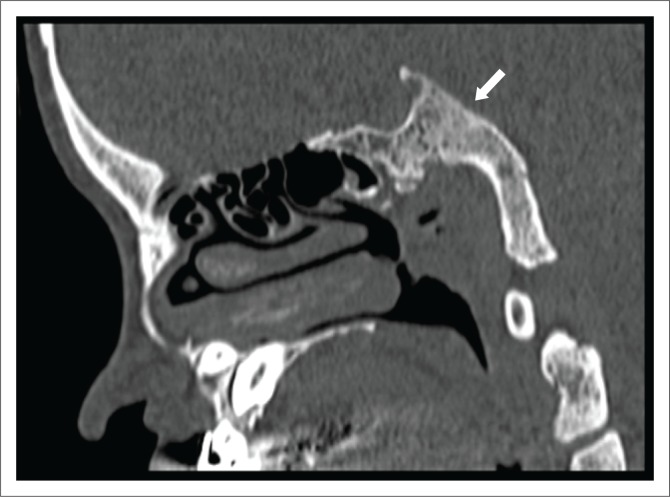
Sagittal-section computed tomography scan shows a dysplastic clivus with its flexion deformity and convexity towards the endocranium (arrow).
